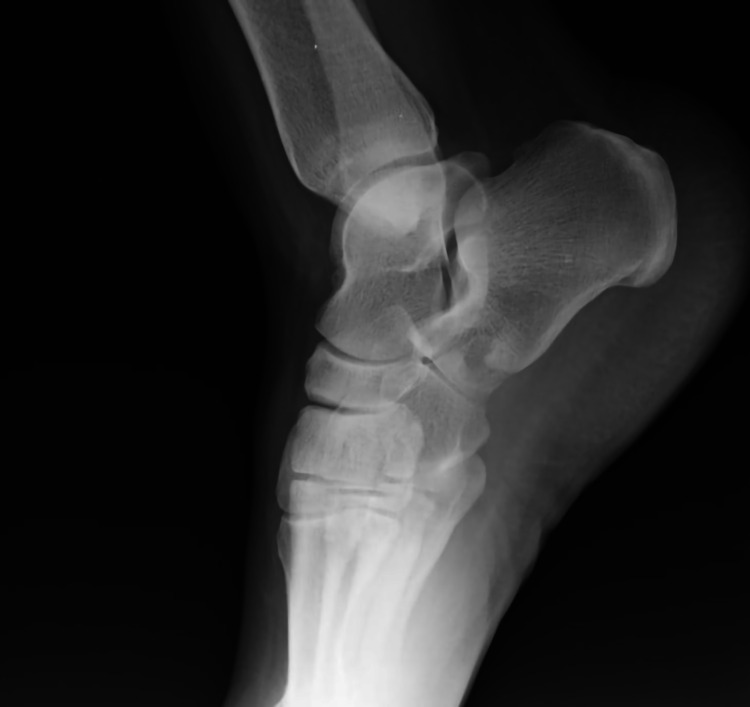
Lateral foot X-ray image taken at 18 months after surgery, in which normal trabecular pattern is seen in the graft region.
